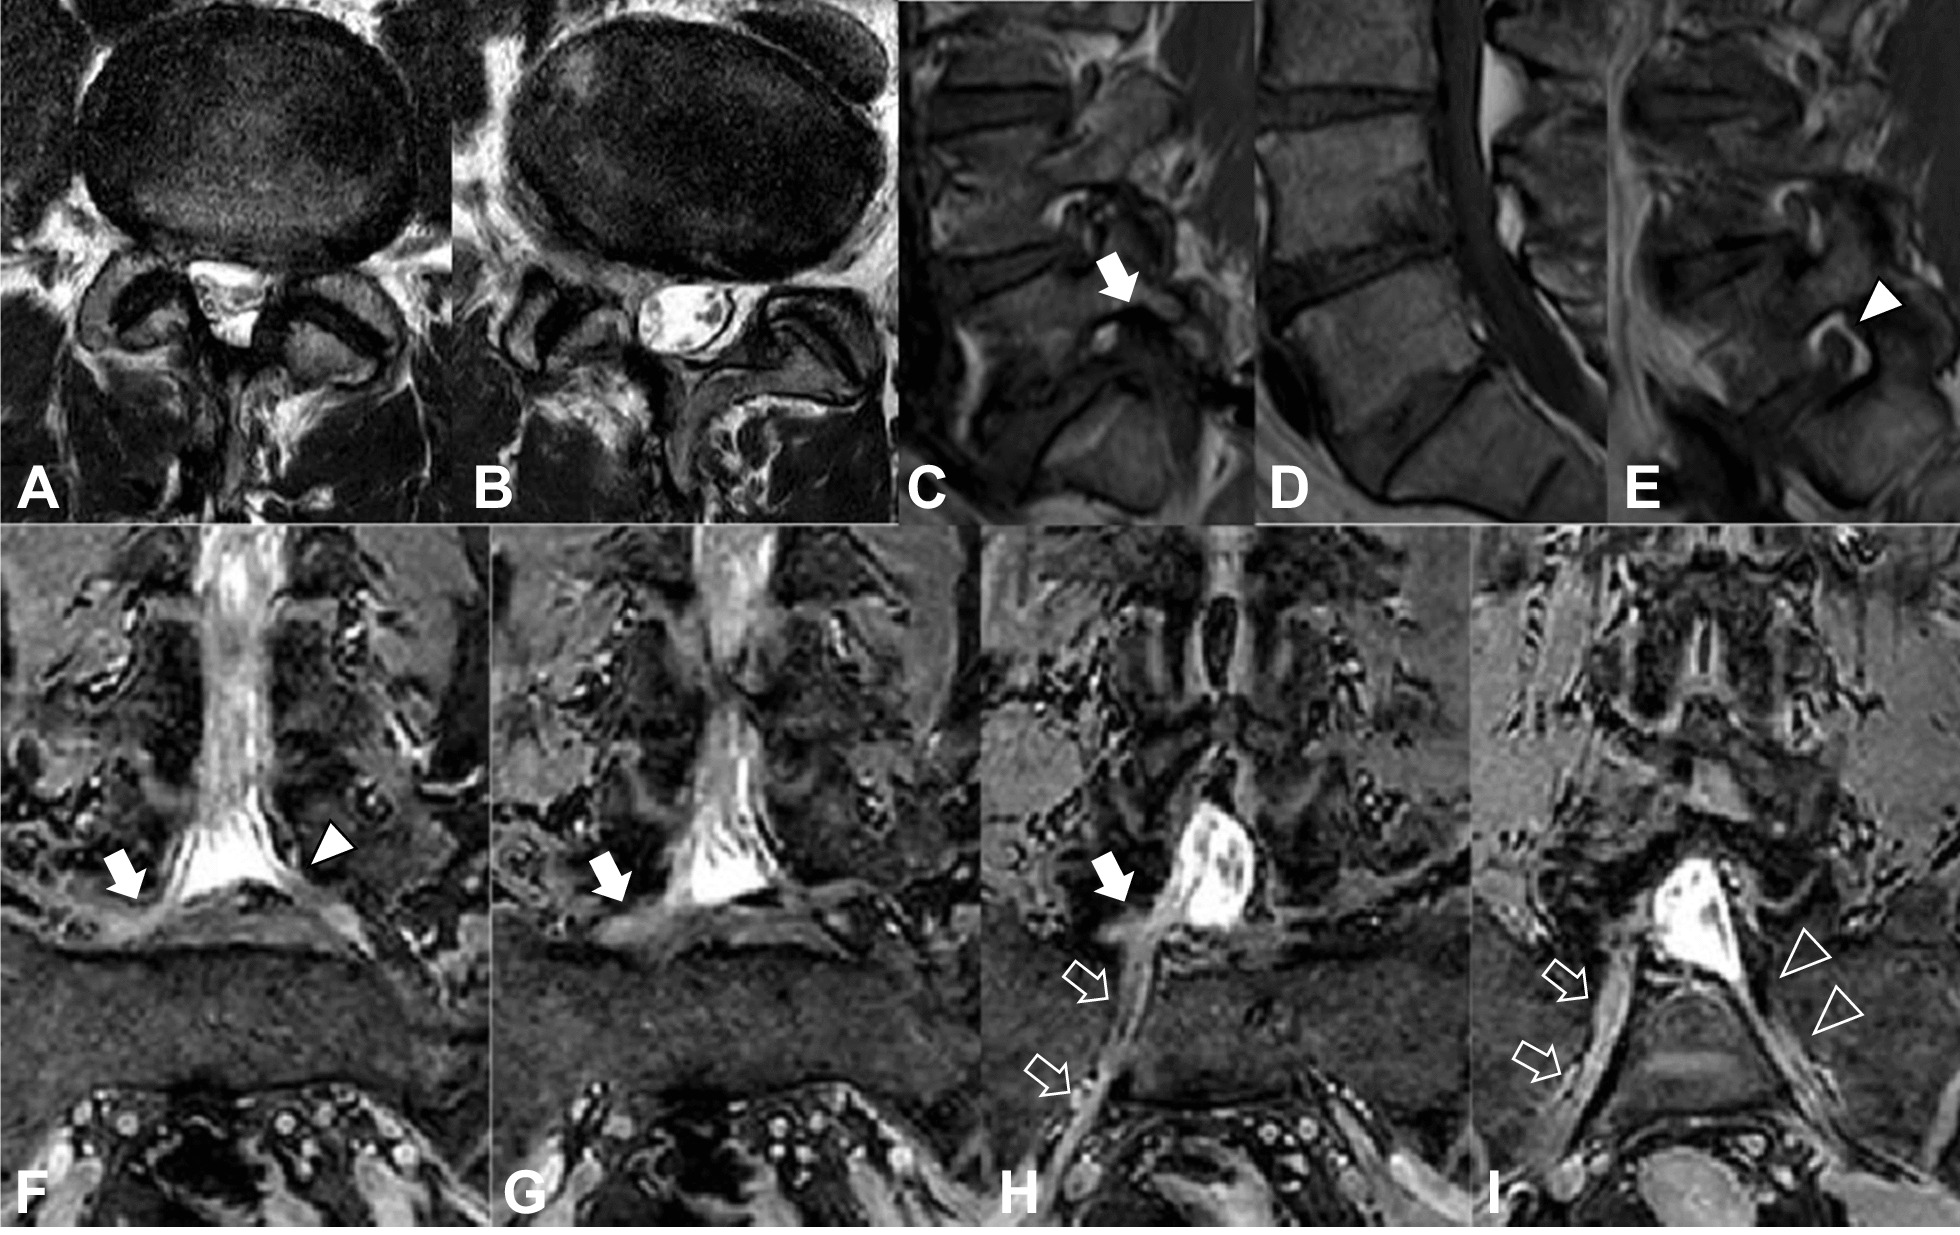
MRI showing no disc herniation on T2-weighted imaging (a L4–5 axial; b L5–S axial), right L5–S foraminal stenosis on T1-weighted imaging (c right sagittal; d central sagittal; e left sagittal), and right CNR (white solid arrow and white outline arrow) on T2-weighted imaging (f–i coronal). The right CNR (white solid arrow) is compressed due to right foraminal stenosis resulting from L5–S disc degeneration. *CNR* conjoined nerve root; *MRI* magnetic resonance imaging
